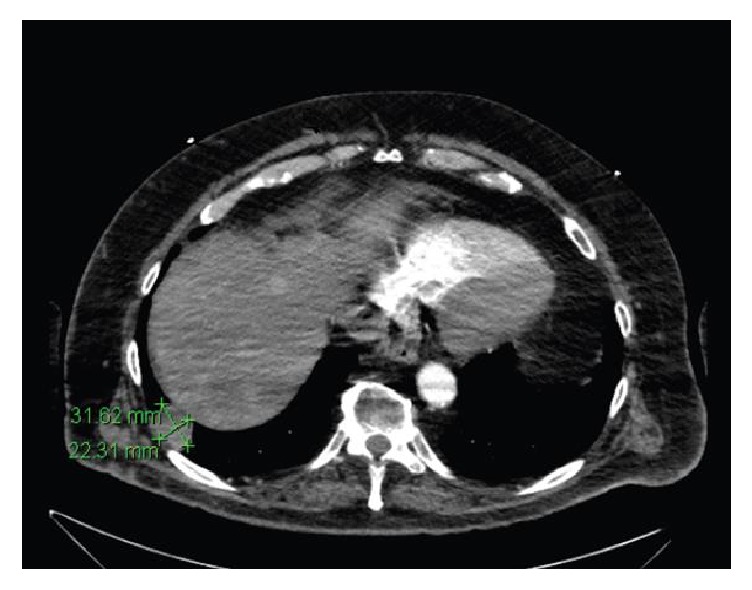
Computed tomography angiography of the thorax showing well-circumscribed, ovoid “cannonball” costophrenic mass in right lower lung lobe, consistent with appearance of metastasis.
